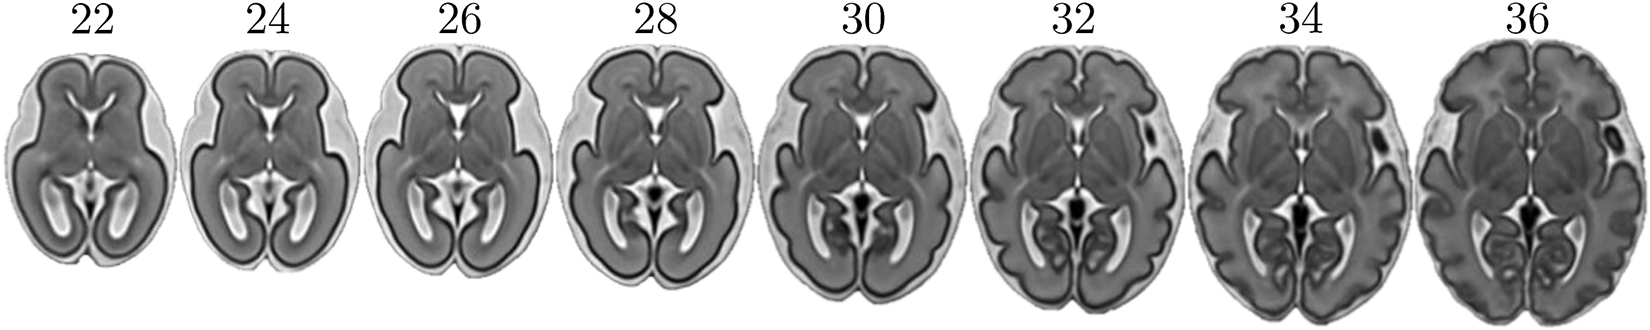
T2-weighted images of the fetal brain covering weeks 22 to 36. The maps are generated from the Uus et al. (2023) publicly available atlas.
